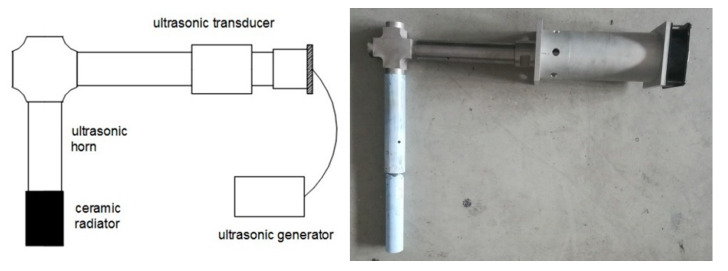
L-Shaped ultrasonic rod device.
